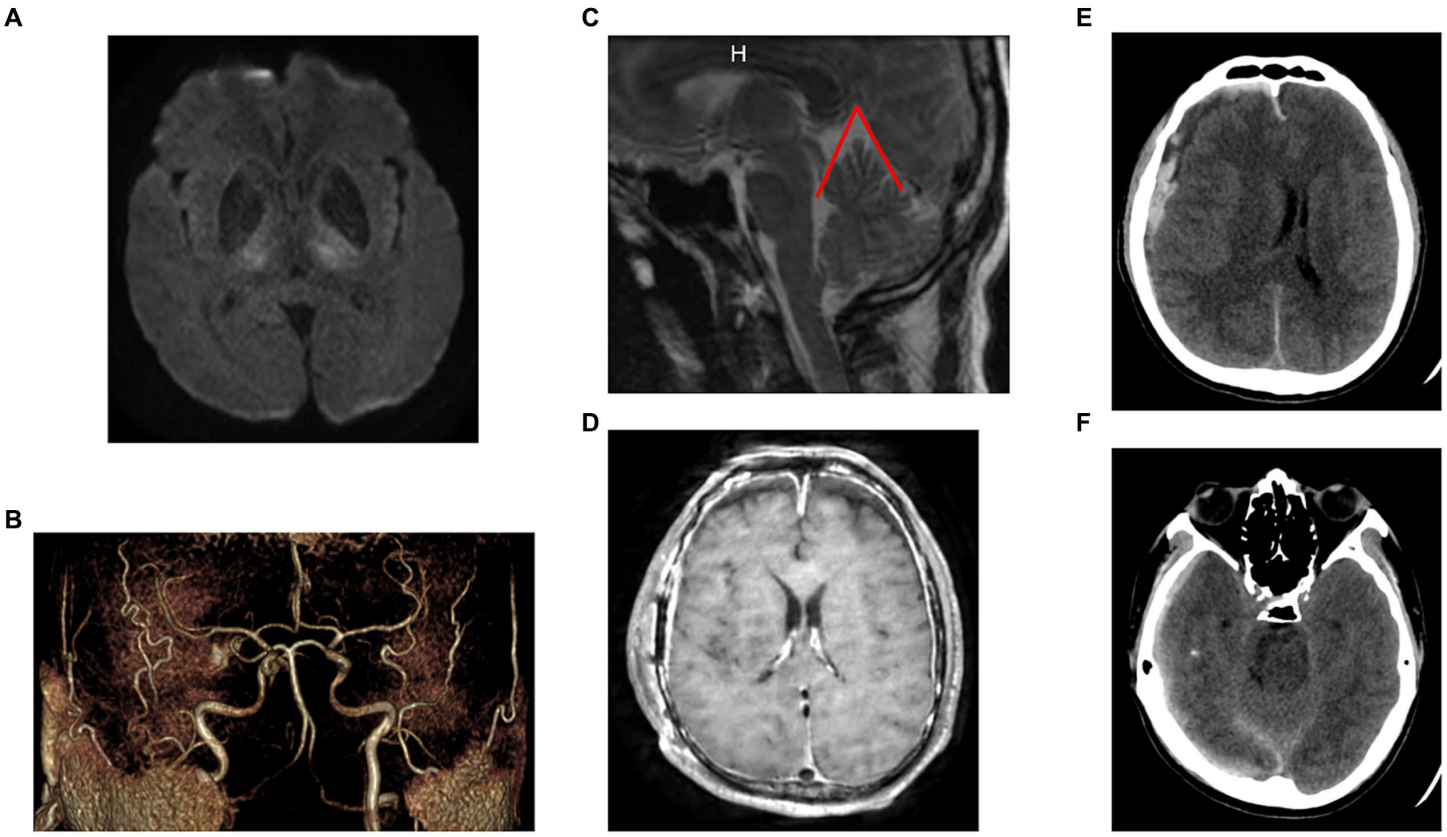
Initial imaging. (A) Initial [day 4 (D4)] MRI (axial diffusion b2000 sequence): bilateral thalamic ischemia. (B) Initial (D4) MRI (3D arterial time-of-flight image): no arterial amputation favoring ischemic stroke. (C) Initial (D4) MRI (sagittal view of a T2-weighted sequence): evidence of posterior sagging of the brain and brainstem, with closure of the angle between the protuberance and the diencephalon, verticalization of the cerebellar tent, herniation of the cerebellar amygdala, favoring the SIH diagnosis. The angle between the vein of Galen and the right sinus was measured at 52° (angle marked in red). (D) D17 MRI (axial T1-weighted sequence): significant and diffuse pachymeningeal enhancement after contrast agent injection and markedly distended dural venous sinuses, favoring the SIH diagnosis. (E) D0 CT-scan (no contrast agent). Evidence of a 13-mm-thick heterogenous subdural collection in the right convexity with significant midline deviation. (F) D0 CT-scan: No uncal herniation, favoring no intracranial hypertension (SDH, subacute subdural hematoma; SIH, spontaneous intracranial hypotension; D0: day of neurosurgical evacuation of the SDH).
